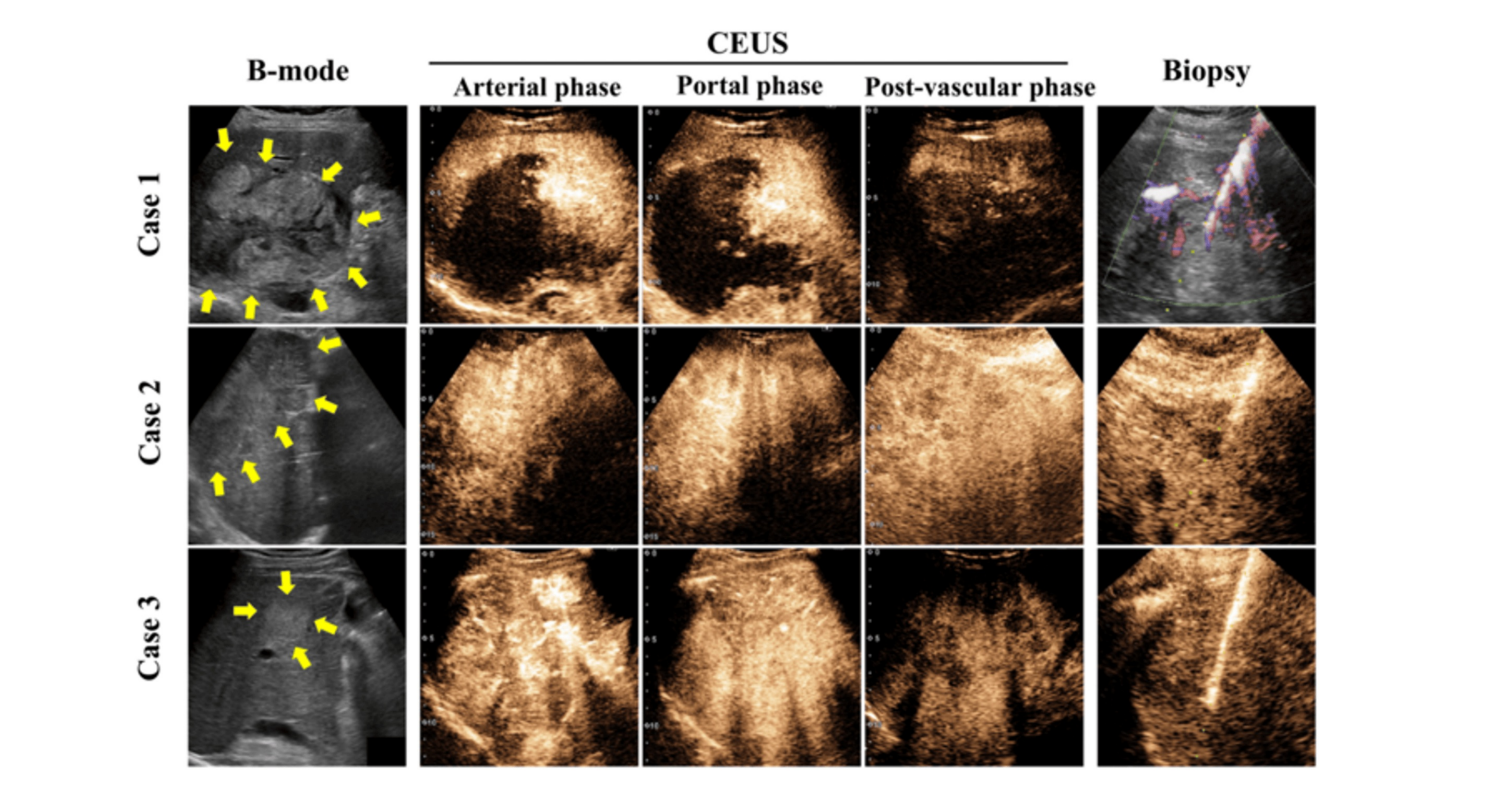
B-mode and contrast-enhanced ultrasound (CEUS) images B-mode and contrast-enhanced ultrasound (CEUS) images in each of the three patients. Images are shown for the arterial phase (15 seconds), portal venous phase (90 seconds), post-vascular phase (15 minutes), and during CEUS-guided percutaneous liver tumor biopsy.
